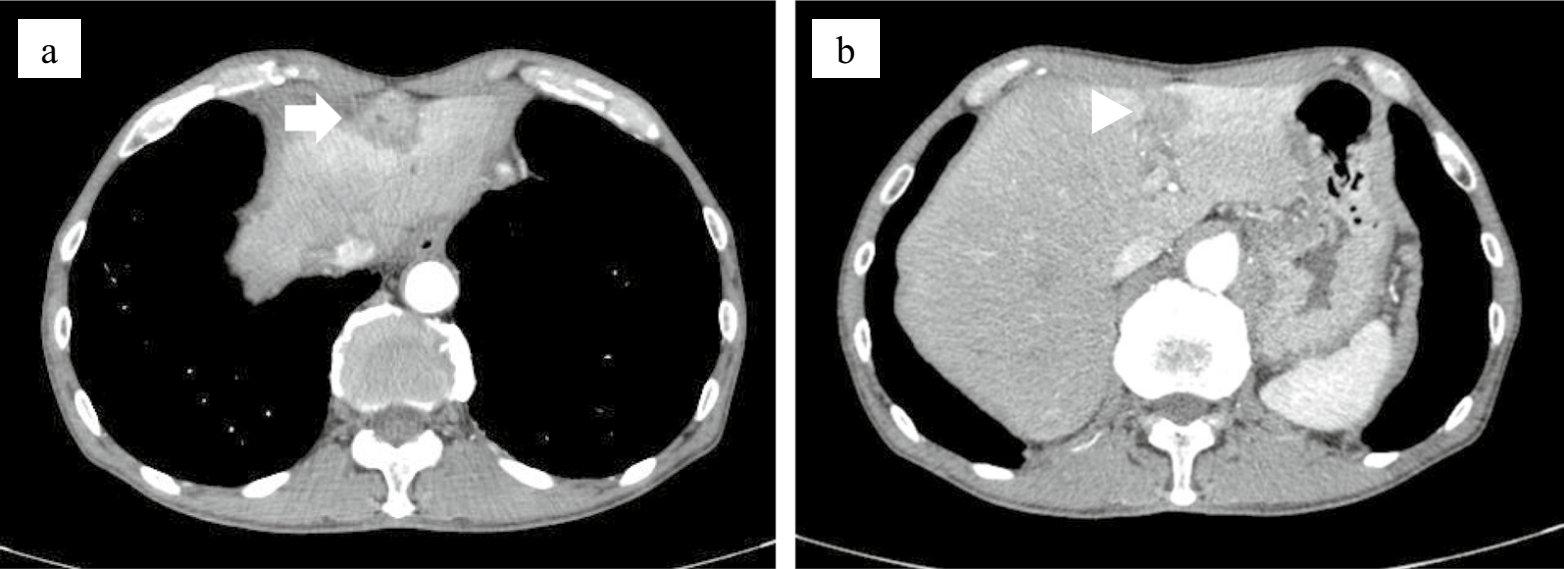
Computed tomography a month following the first discharge. a The falciform ligament mass (white arrow) had increased in size, and the border between the mass and the liver was obscure. b A low-density nodule had emerged in liver segment three (white arrowhead)
